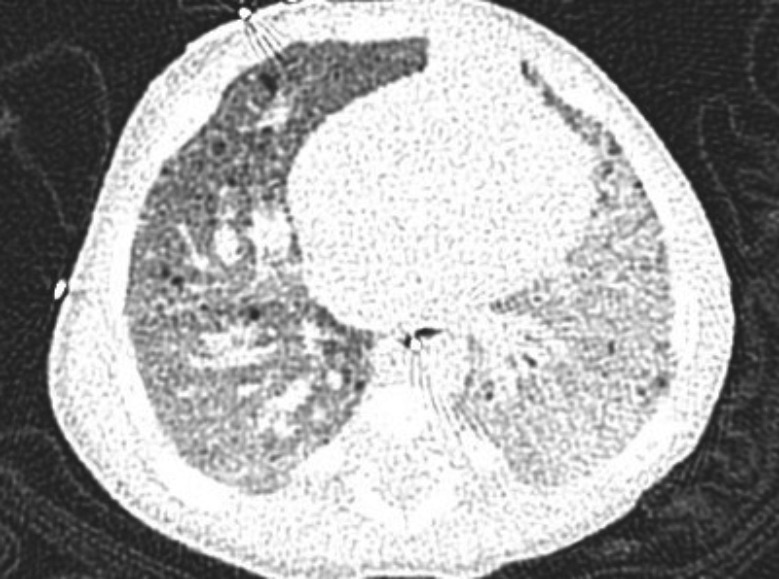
CTA diffuse interstitial reticular markings with multifocal opacities
